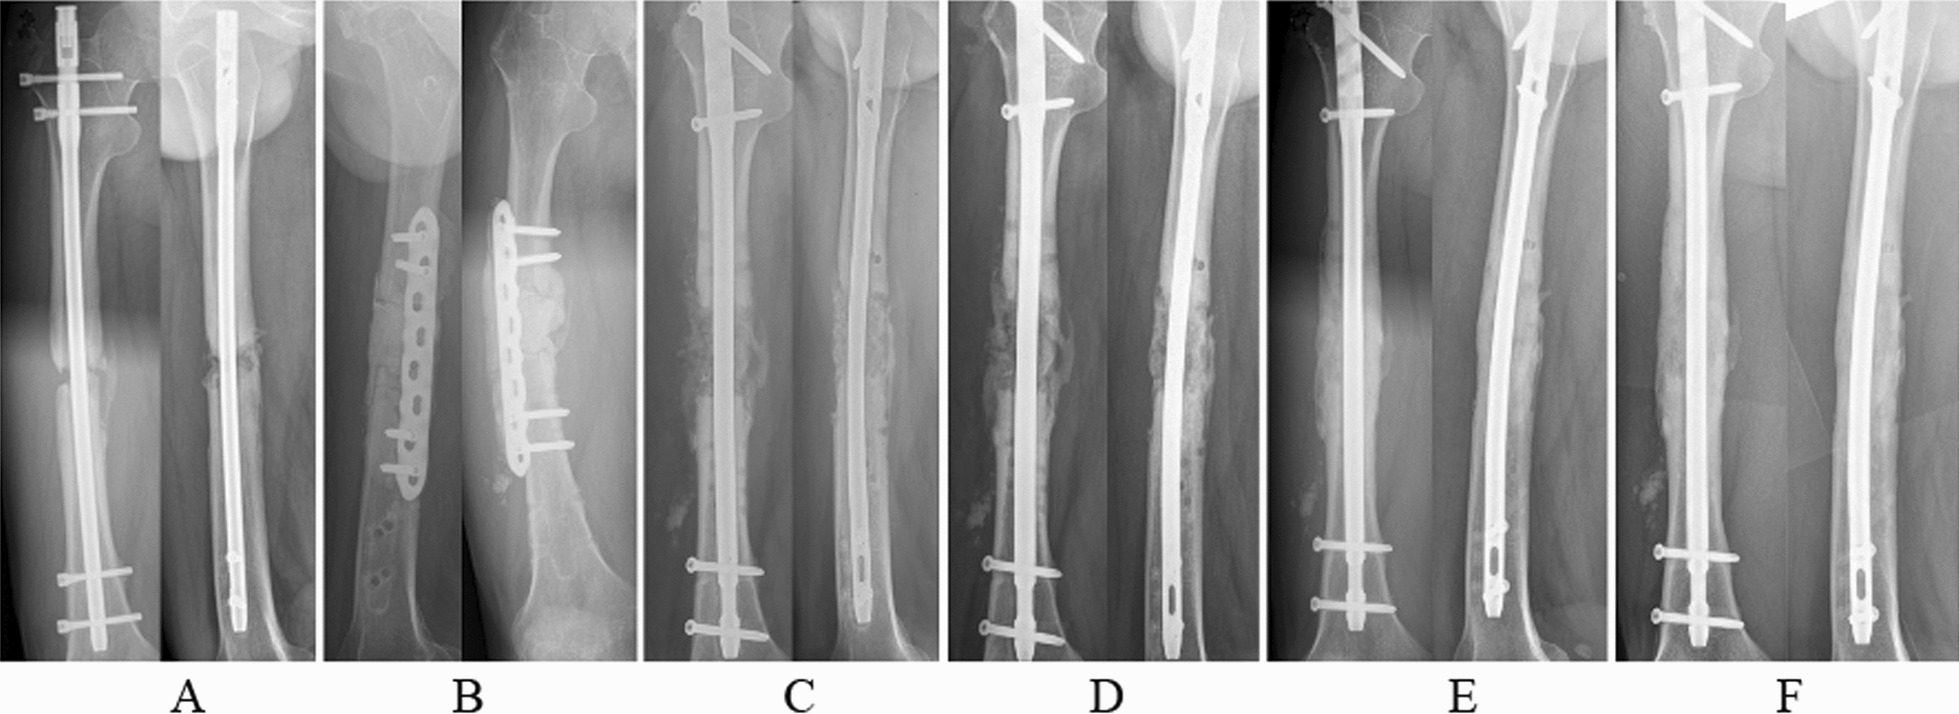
A male patient (28 years old) with bone nonunion in the right femur was treated with the staged method of the induced membrane technique (IMT), and a low-toxicity infection (coagulase-negative Staphylococcus spp.) was detected after surgery. A Preoperative radiographs showed less callus formation at the fracture site after initial osteosynthesis. B Debridement and bone gap filled with antibiotic bone cement. C–F Radiographs at 2, 6, 12, and 18 months after IMT treatment. Bone union was achieved at 12 months, and complete bone consolidation was achieved at 18 months
